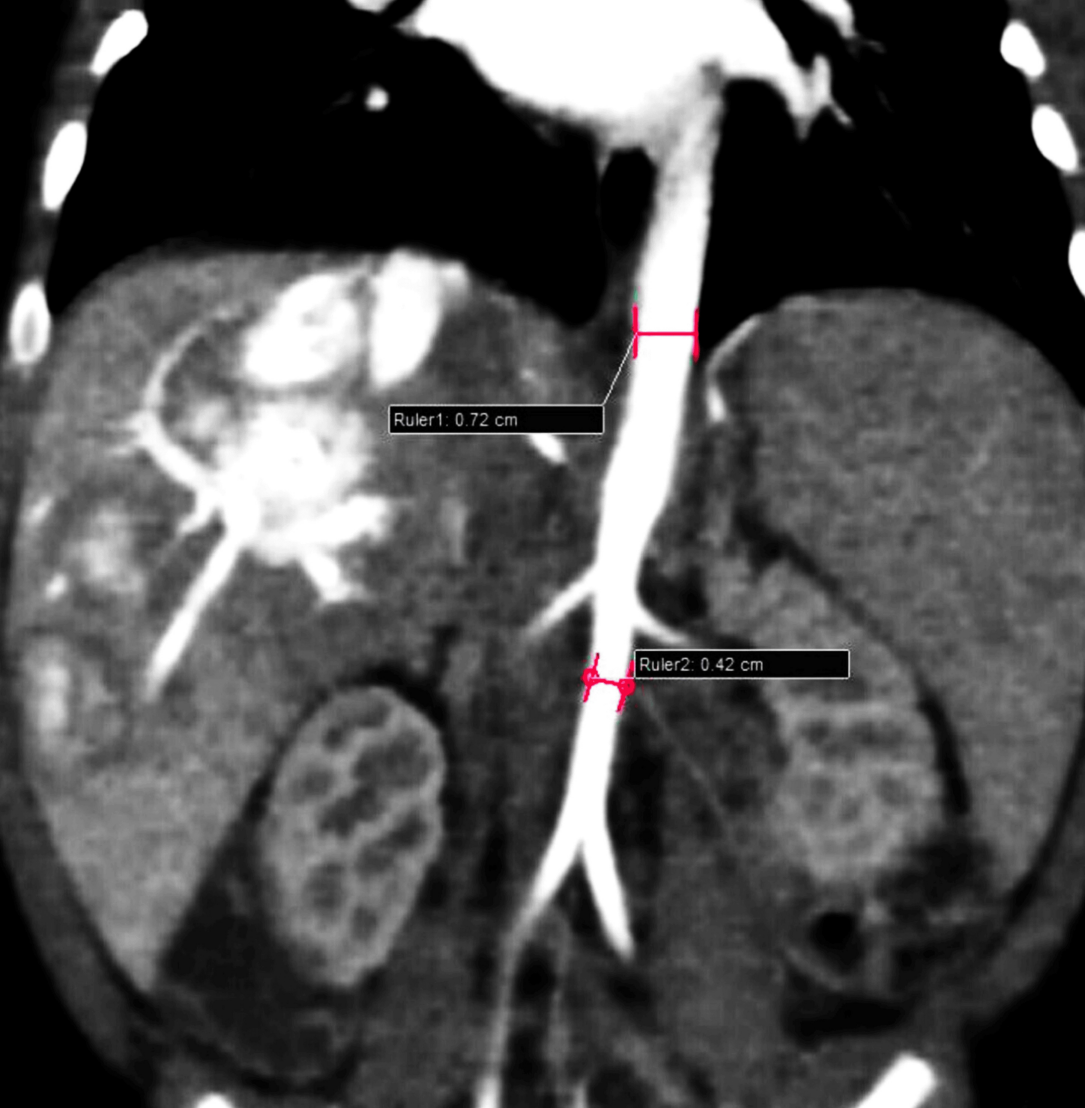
Reformatted coronal section of contrast-enhanced CT image showing narrowing (pink marking) of the aorta distal to celiac trunk - suggestive of mid-aortic syndrome CT: computed tomography
